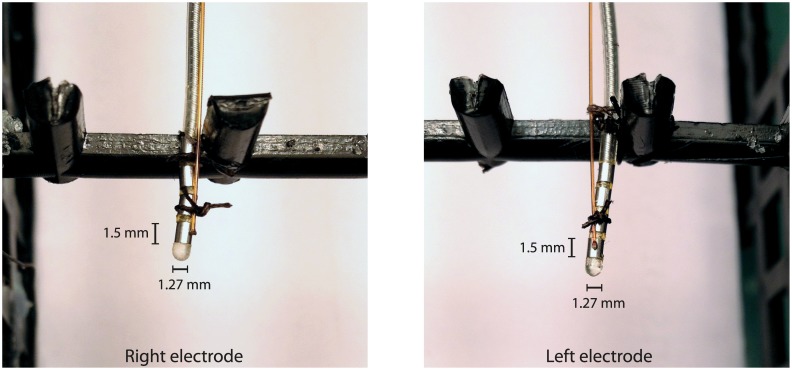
The fibre-optic temperature sensors positioned at the distal electrode contacts. The fine golden leads are the temperature probe optical fibres, whereas the grey leads with 4 visible metal contacts are the DBS electrode leads. Suture silk was used to ensure that the thermometer probes were in close thermal contact with the distal electrode contact surfaces, previously demonstrated to be the sites of greatest MRI-induced heating.
